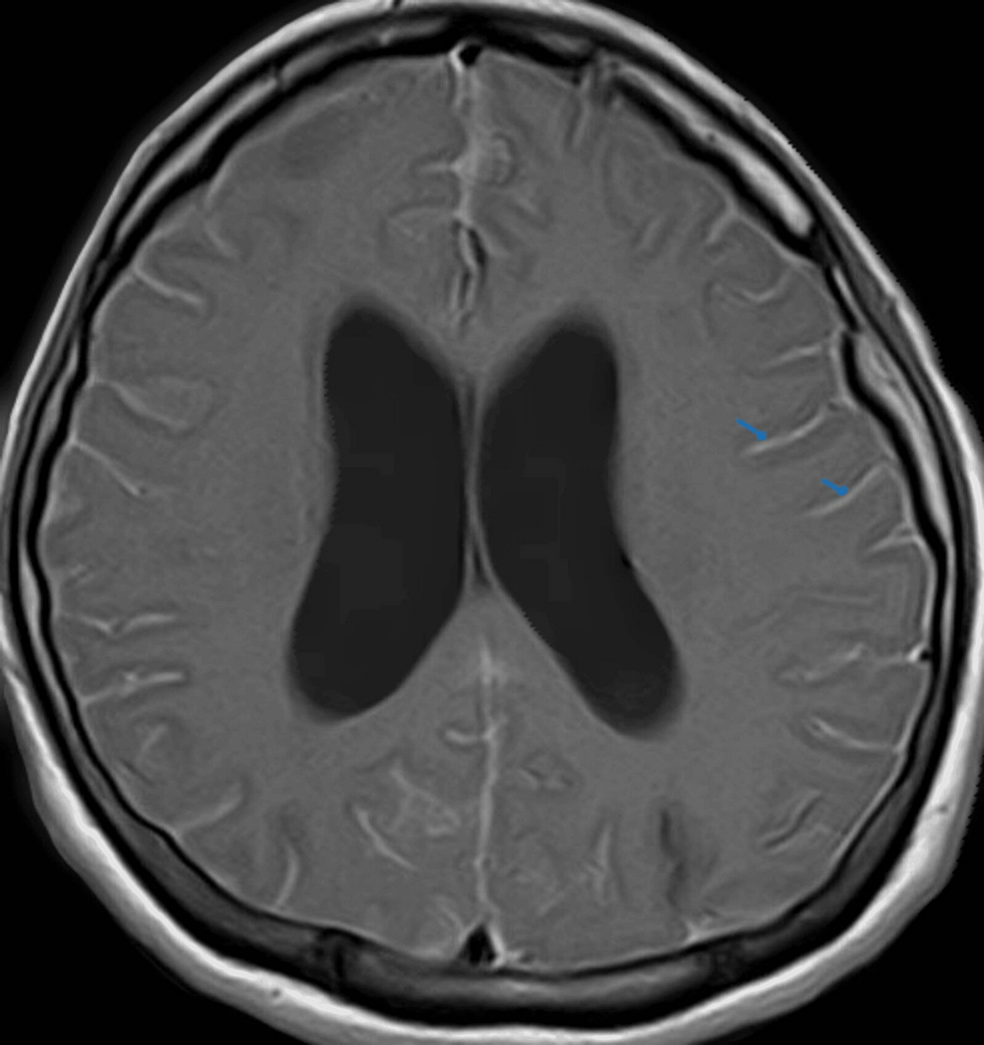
Gadolinium-enhanced T1-weighted brain MRI (axial view) after a year of follow-up shows diffuse leptomeningeal enhancement (blue arrow).
